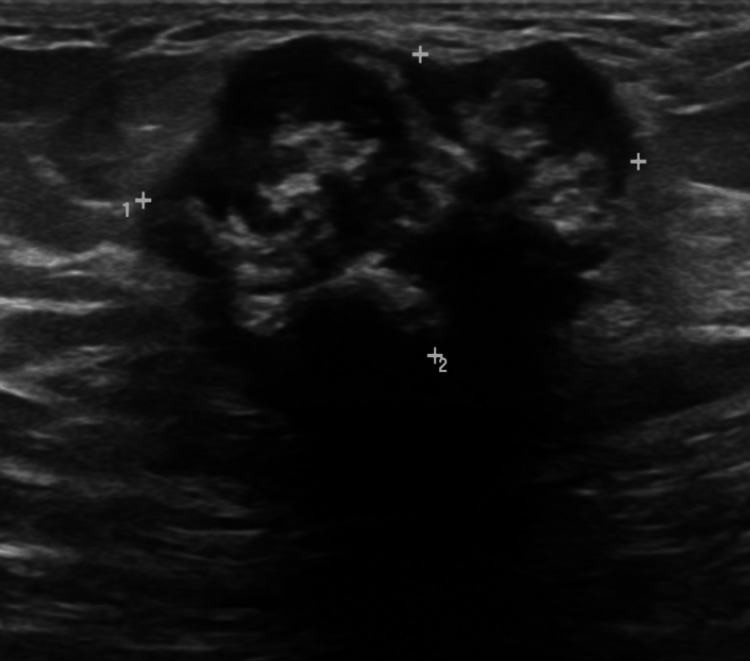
Targeted ultrasound of the left breast demonstrates a hypoechoic mass measuring 3 × 1.8 × 2.7 cm at two o’clock, 6 cm from the nipple, with lobulated margins, dense calcifications, and posterior shadowing (BI-RADS 4A).
